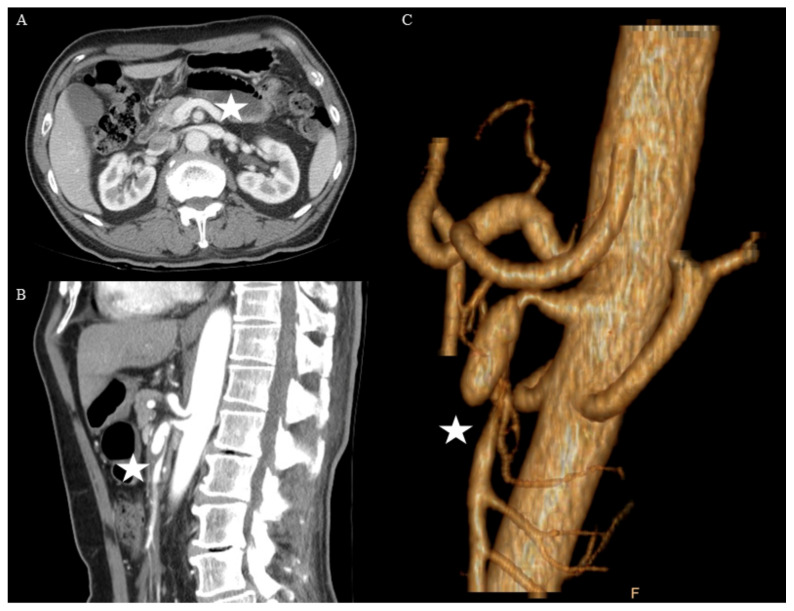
This was a 66-year-old man with Yun’s IIa spontaneous isolated dissection of the superior mesenteric artery (asterisks). Computed tomography (CT) scans, (A) axial, (B) sagittal, and (C) three-dimensional, demonstrated small patent true lumen, and conservative treatment was applied first.
